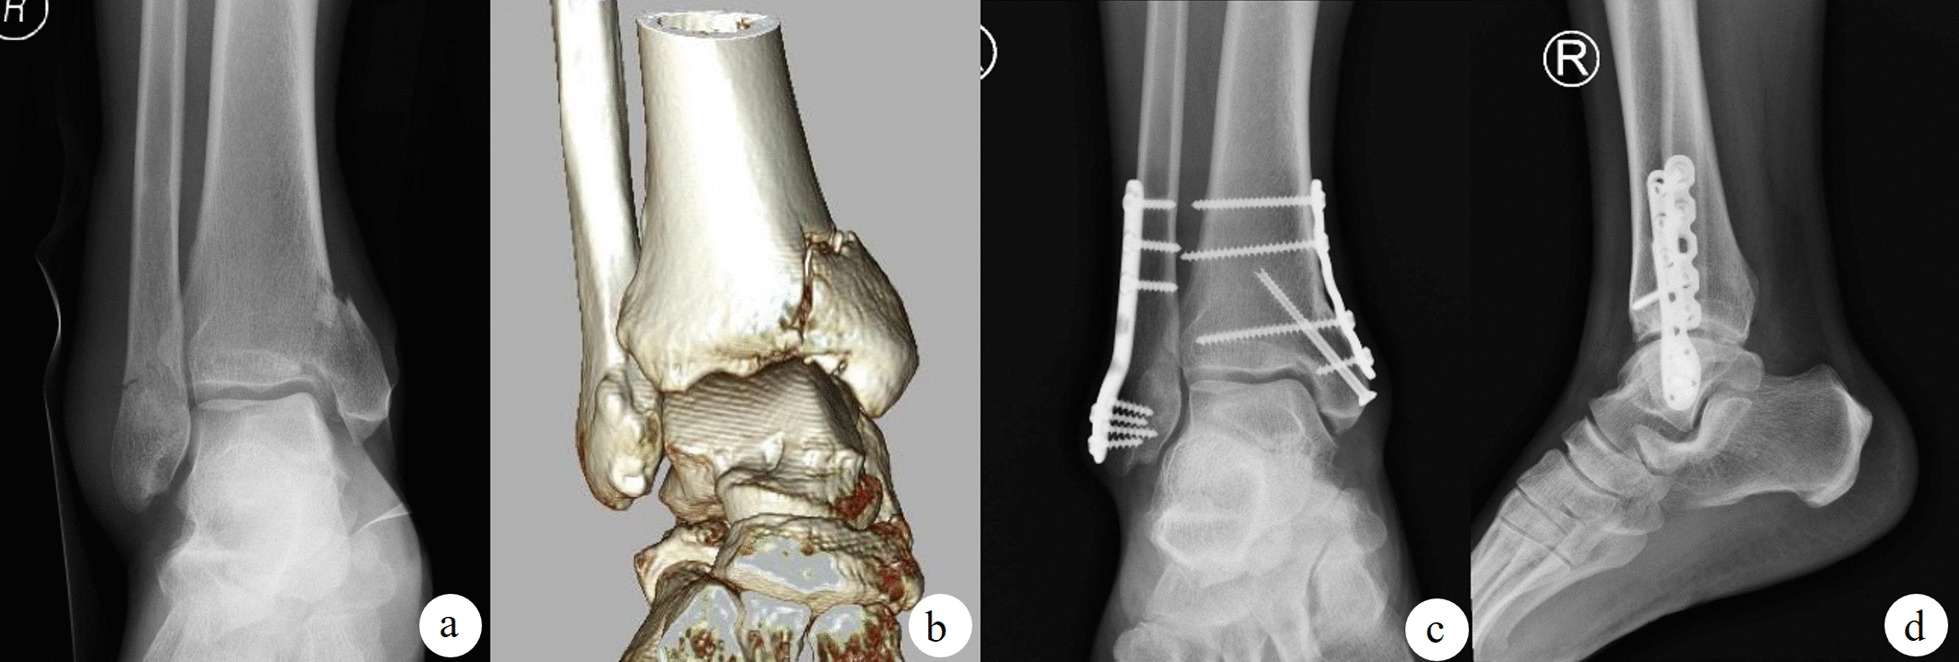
The use of buttress steel plates to assist screw fixation can eliminate the disadvantages of insufficient distal fixation. a The orthographic X-ray of the fracture; b the three-dimensional CT reconstruction after the fracture, c, d the orthographic X-ray of the Buttress plate combined with screw internal fixation
